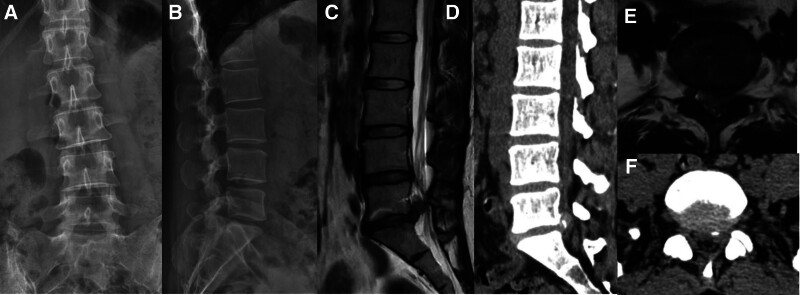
Patient preoperative radiologic images. (A, B) Preoperative anteroposterior and lateral radiographs. (C) Preoperative parasagittal T2-weighted magnetic resonance imaging (MRI) image. (D) Preoperative sagittal computed tomography (CT) image. (E) Preoperative axial T2-weighted MRI image. (F) Preoperative axial CT image.
